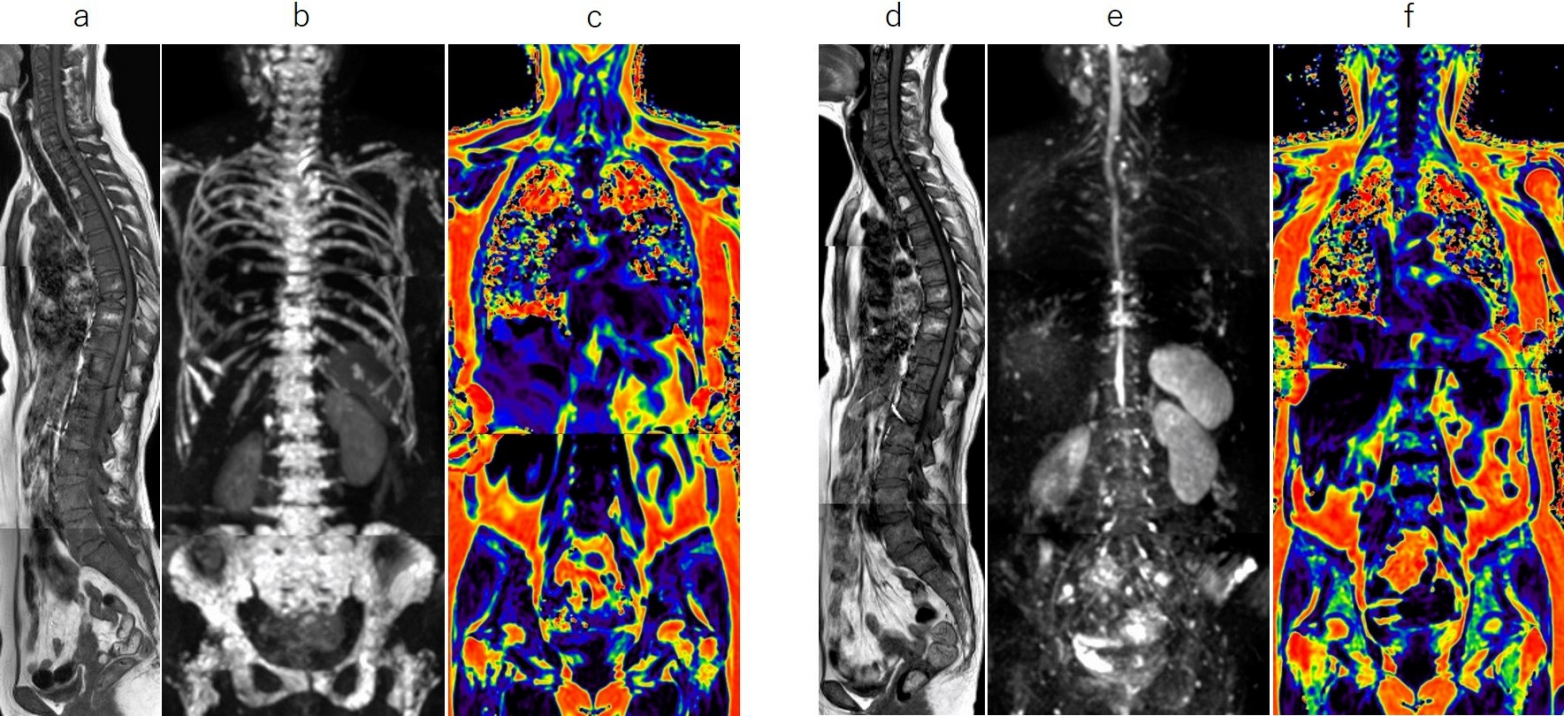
Whole-body MRI with a diffuse-dominant pattern. Sagittal T1-weighted images (T1WI, a), whole-body diffusion-weighted MRI (WB-DWI, b), and fat fraction (FF) map (c) at baseline, and corresponding T1WI (d), WB-DWI (e), and FF map (f) at 2 cycles of chemotherapy for a 60-year-old woman with symptomatic myeloma who relapsed after autologous stem cell transplantation. MRI shows diffuse low signal in the spine on T1WI (a) and diffuse high signal in the axial skeleton on WB-DWI (b) at baseline. After 2 cycles of chemotherapy with carfilzomib, lenalidomide, and dexamethasone, total diffusion volume (tDV) has significantly decreased (from 201 ml to 21 ml) and the FF (f) in the lumbar bone marrow has significantly increased (from 2% to 25%), indicating a decrease in the tumor mass.
